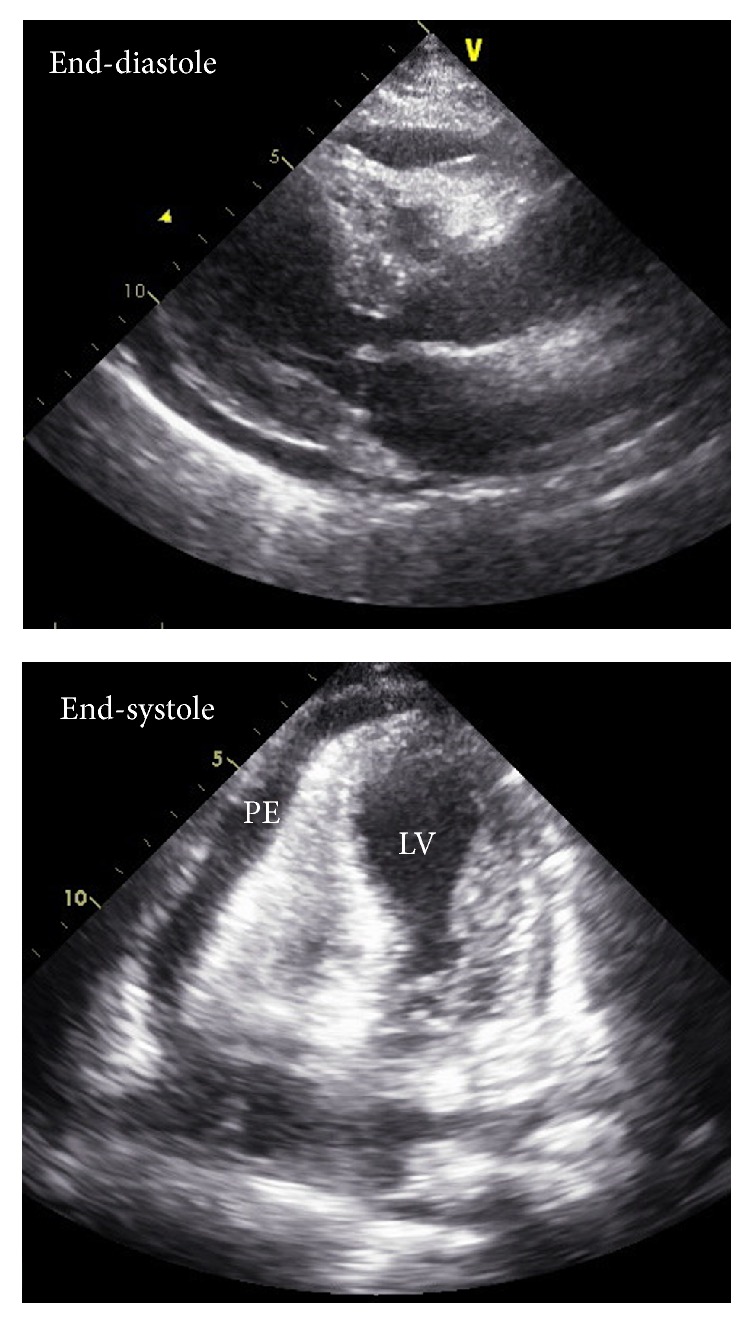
Transthoracic echocardiography showed small amounts of pericardial effusion, apical ballooning, and right ventricular collapse at end-diastole.
